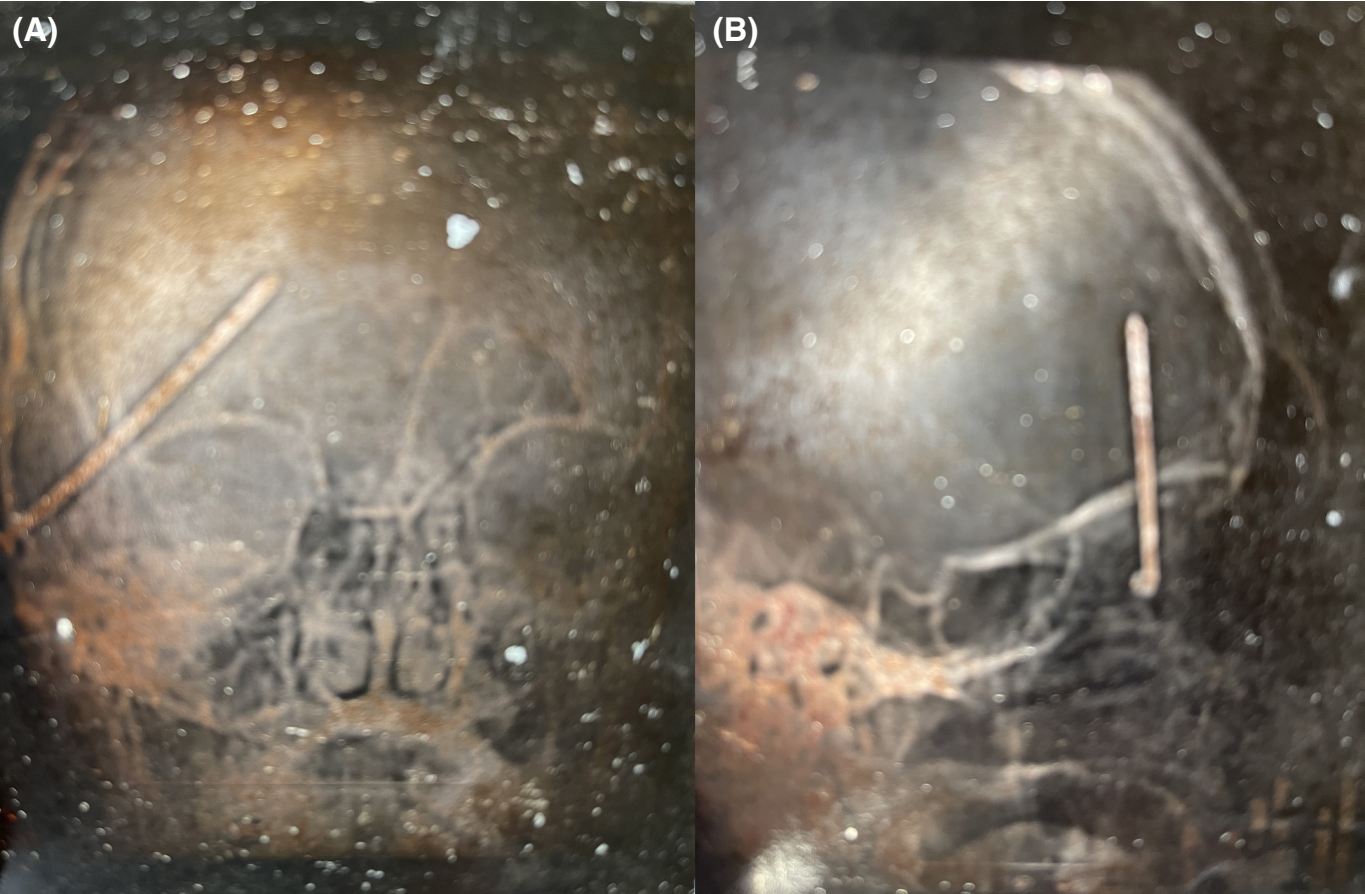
Anteroposterior (A) and lateral (B) radiographs reveal the superomedial trajectory of the nail into the brain parenchyma. The patient had been carrying the radiograph prints in his wallet for twenty years
